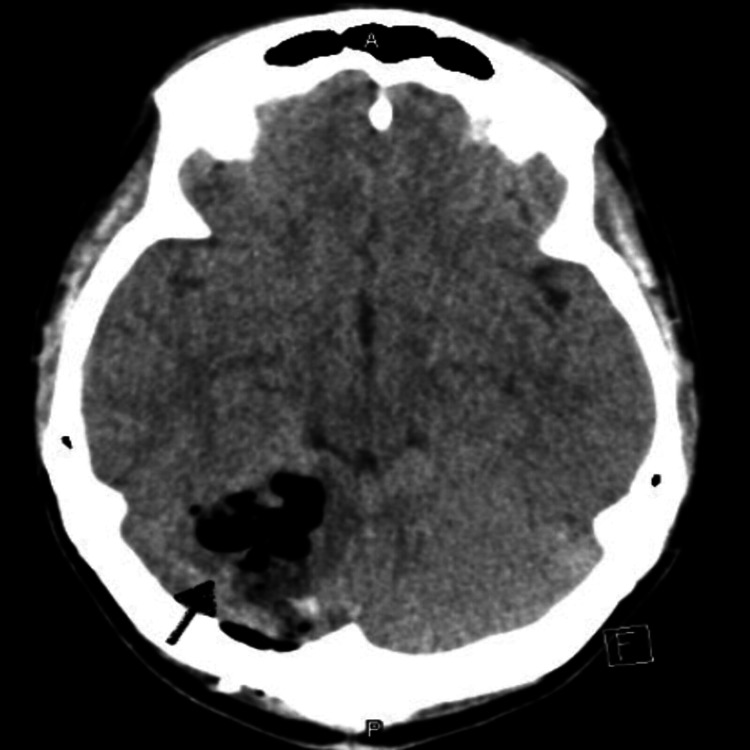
CT without contrast of head after the second resection of the tumor.
